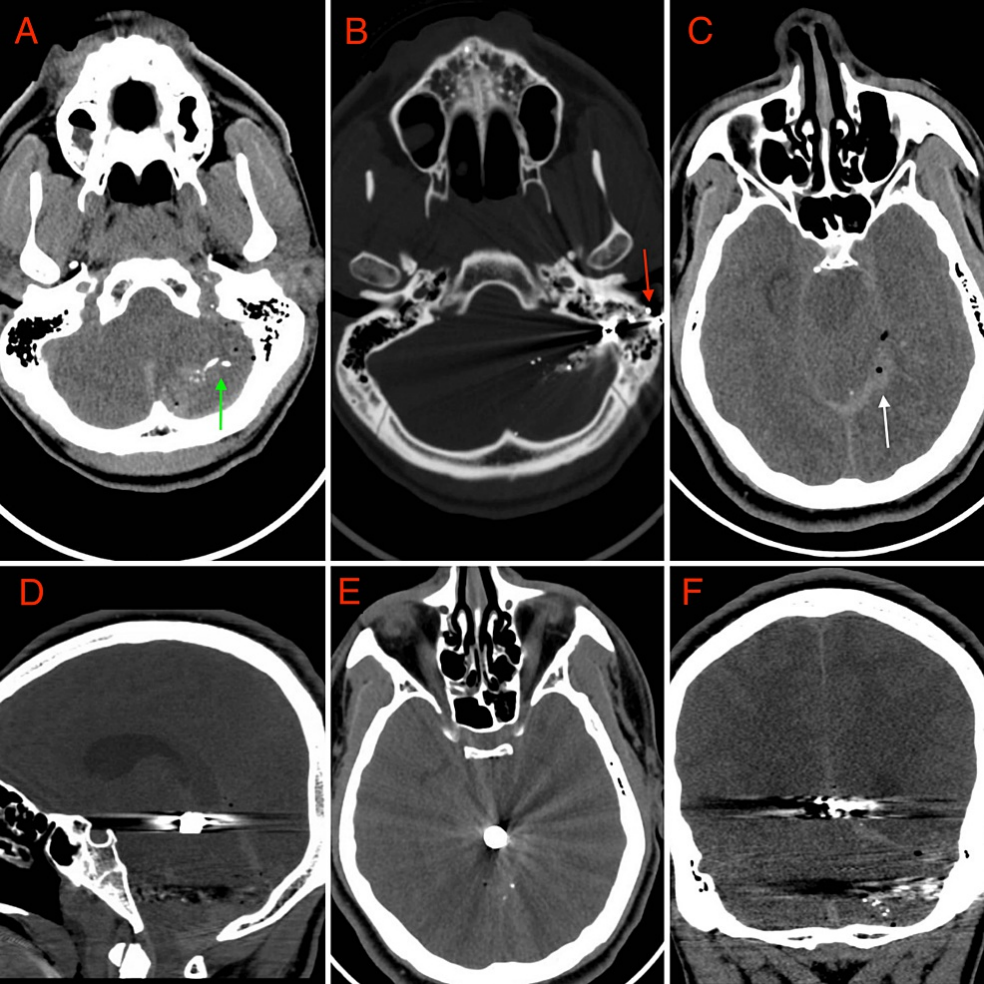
Initial diagnostic imaging following emergency endotracheal intubation (A-F) 1A-B: Axial computed tomography (CT) images showing the bullet’s entrance wound (red arrow) through the left petrous bone with bone fragments and shrapnel in the cerebellum (green arrow); 1C: Axial CT image showing pneumocephalus and hemorrhage (white arrow) along the left tentorial leaflet, demarcating the path of the bullet; 1D-F: sagittal (D), axial (E), and coronal (F) CT images showing bullet rested in the quadrigeminal cistern directly posterior to the midbrain.
